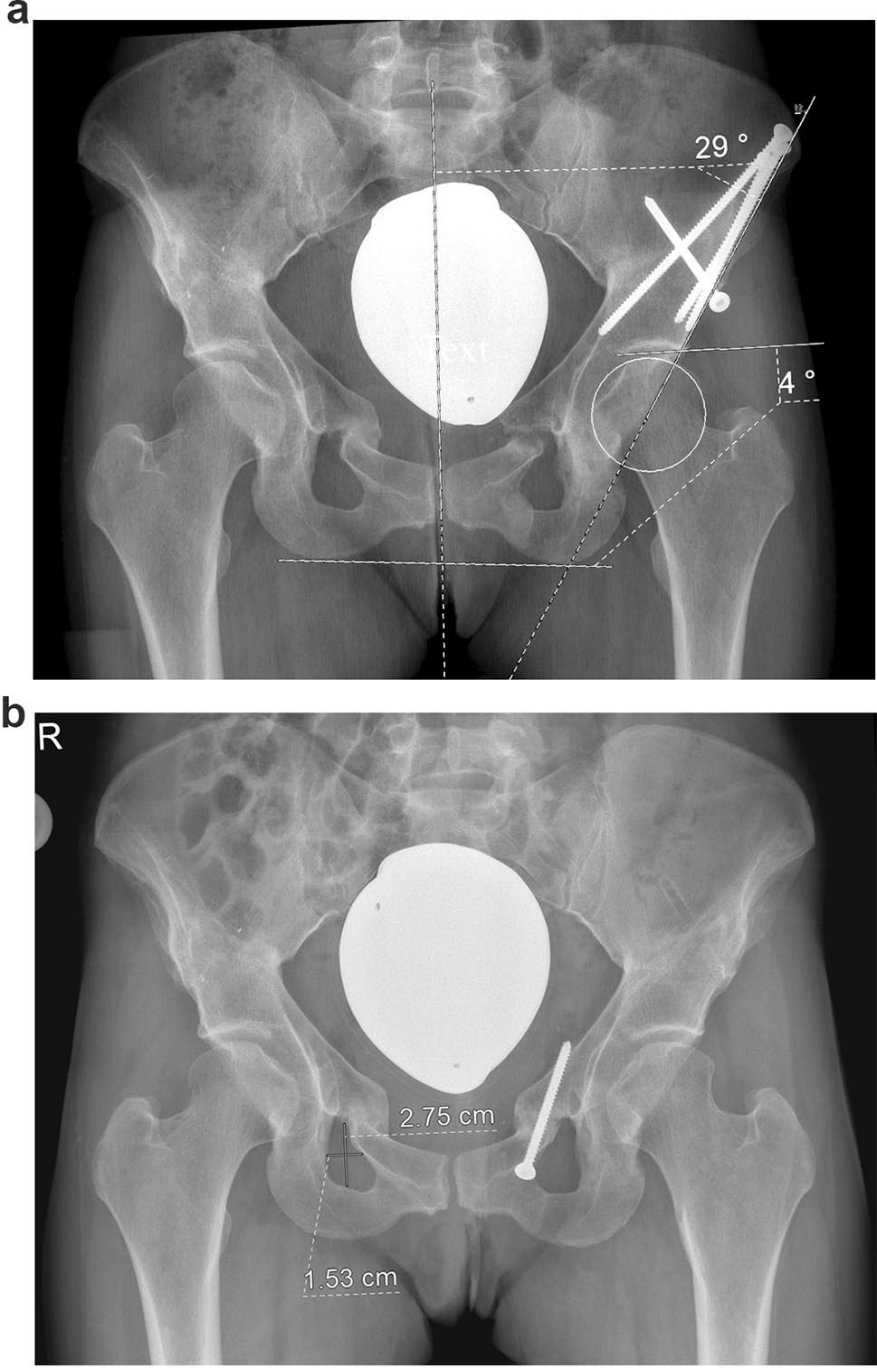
a Pelvic radiograph of a female, performed one year after TPO. Surgery around the left hip took place at the age of 38 years, the right hip had been treated two years earlier. BMI was 20.5, the patient consumed 20 cigarettes a day. TPO was indicated due to symptomatic hip dysplasia with an LCEA of 14° and an AI of 18°. A rather large acetabular correction had to be performed, resulting in an LCEA of 29° and an AI of 4° (Δ LCEA pre- to postoperative: 15°, Δ AI pre- to postoperative: 14°). The patient complained about groin pain, different from the joint-related pain she experienced before TPO. A test infiltration of the left hip joint did not relieve the pain, thus the non-union of the pubic osteotomy was considered the cause of the complaints. The measurement routine of LCEA and AI is displayed at the left hip. Please see the explanation in detail in the main text under the heading “Measurement routine and definition of a non-union”. b Revision surgery of the pubic osteotomy was performed 1 year after TPO, simultaneously with the removal of the fixation screws from the ileum, including autogenous cancellous bone grafting from the iliac crest and osteosynthesis of the pubic bone with a fully threaded lag screw. The assessment of the height-width relation is demonstrated on the right obturator foramen. Please see the explanation of the measurement routine in the main text under the heading “Radiographic management and follow-up regimen”
